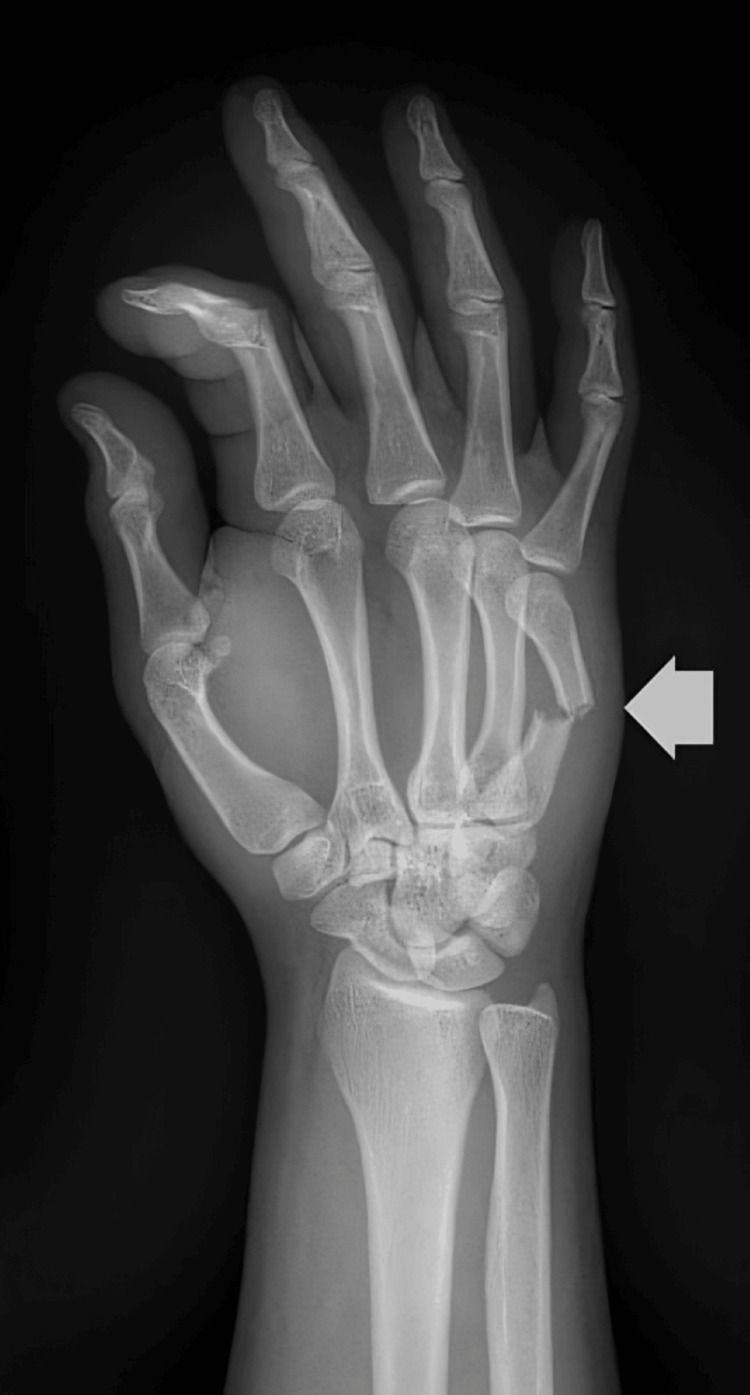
Radiographic image of the right hand. The white arrow highlights a fracture of the shaft of the fifth metacarpal.
